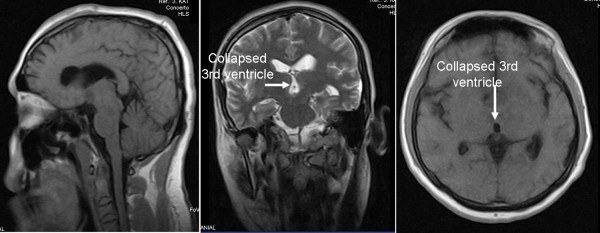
A follow-up cranial MRI performed six months post-operatively showed no recurrence of lymphoma and collapsed third ventricle.
